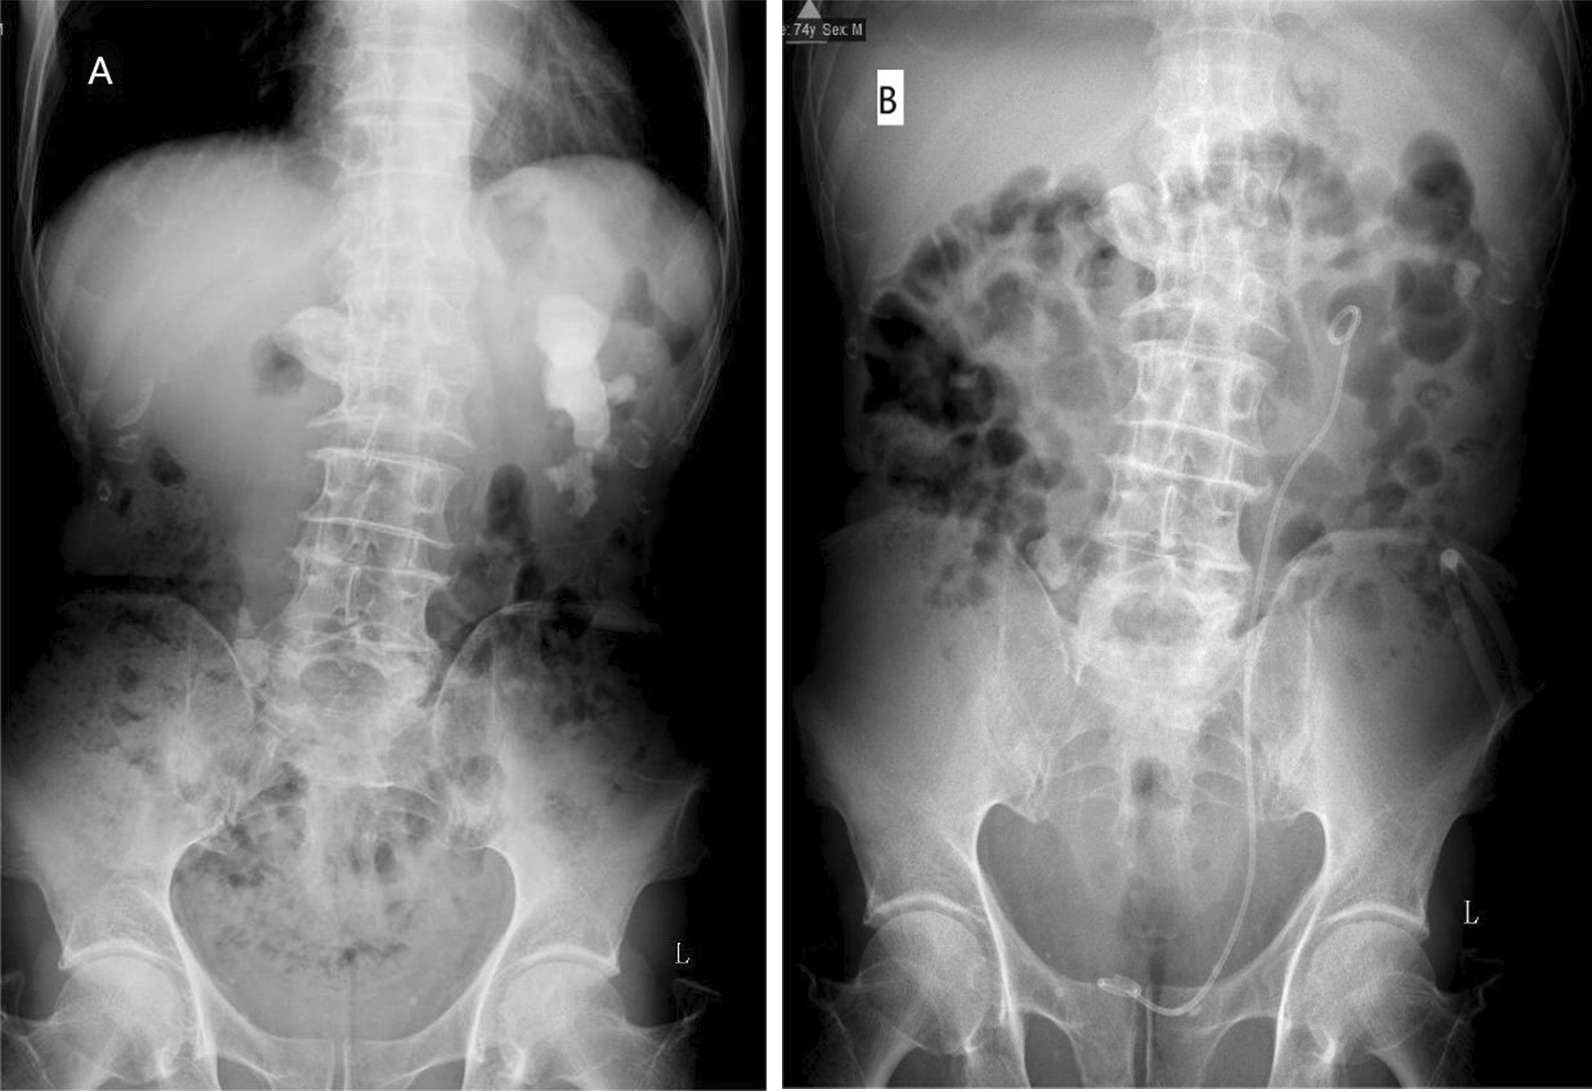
A A 74 year old male with staghorn calculi in the left kidney was treated with single channel, 22F, percutaneous nephrolithotripsy. B Most of the calculi were cleared by KUB after operation, and a small amount of residual calculi were found in the kidney. KUB = Kidney, Ureter, and Bladder X-ray
